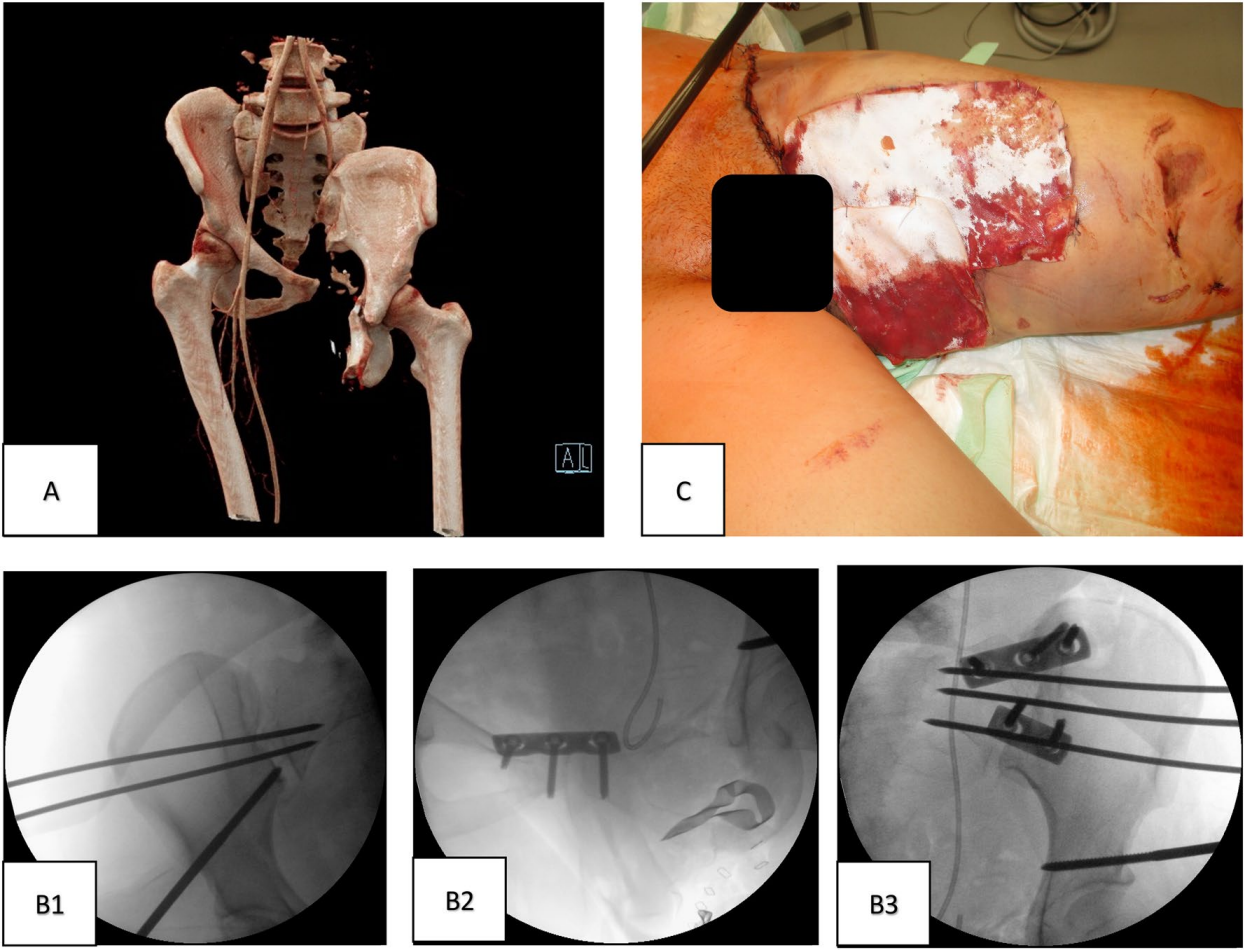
Patient 2 initial surgical strategy. A Initial angio 3D-CT reconstruction of the sustained injury (AO classification: 61-C3.2 a, d, j—left iliosacral disruption—right sacral fracture and 62-A1.2 acetabulum fracture on the right side; complete traumatic hemipelvectomy). B Closed reduction of the pelvis via supraacetabular external fixator and temporary fixation via percutaneous K-wires bilateral (B1, B3) and additional SI-plating on the left side (B3) as well as plate osteosynthesis of the symphysis pubis (B2). C Temporary wound coverage in the groin and medial thigh with Epigard^®^ (Biovision GmbH, Ilmenau Germany)
